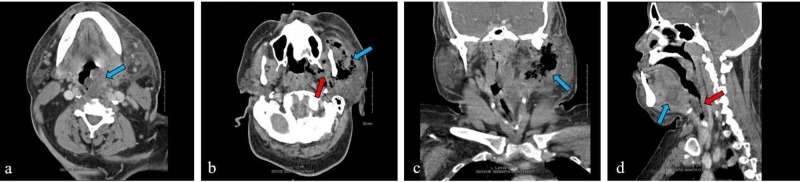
Contrast-enhanced computed tomography of the soft tissues of the neck showed rightward displacement of the hypopharyngeal airway (a, blue arrow, axial image). Also noted were abscesses of the left masticator muscle (b, blue arrow, axial image), medial and lateral pterygoids (b, red arrow, axial image), left stylomandibular canal (c, blue arrow, coronal image), and the sublingual space (d, blue arrow, sagittal image) with narrowing of airway to 4 mm (d, red arrow, sagittal image).
